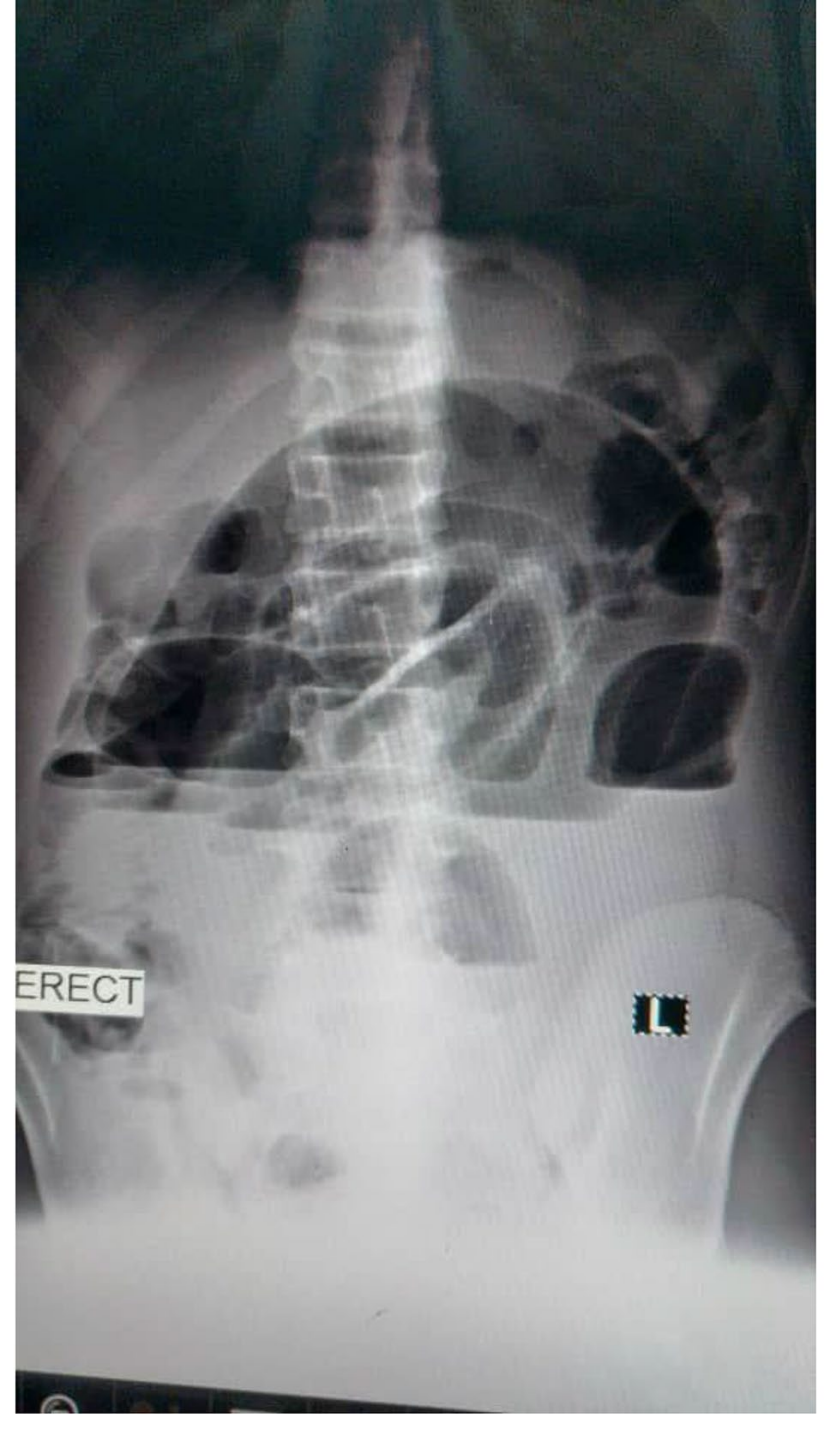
Erect abdominal x-ray revealed multiple air-fluid levels
